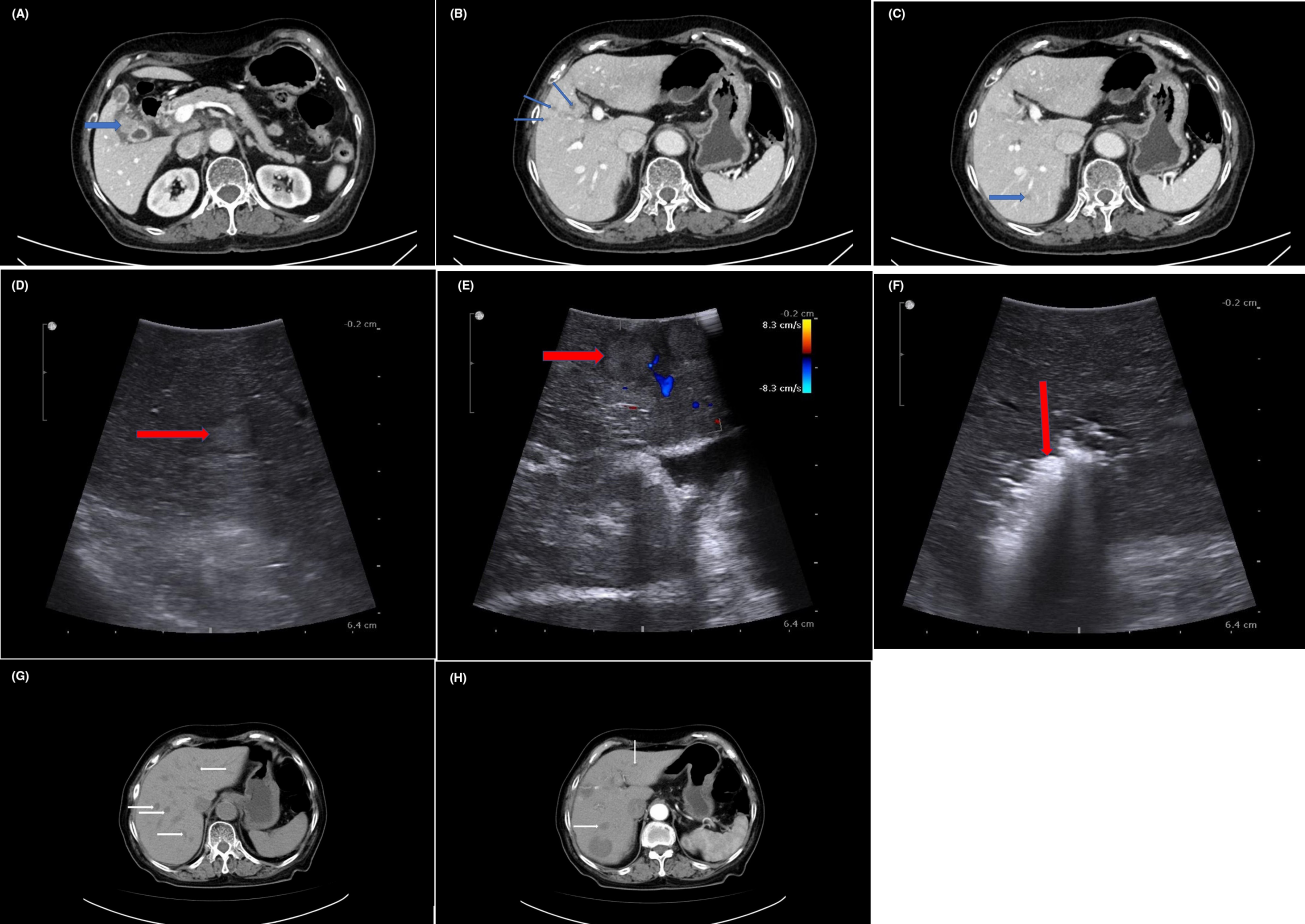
Representative figures of GBC patients with CTMLD. (A) primary gallbladder lesion (blue arrow); (B) regional metastatic liver lesions (blue arrow); (C) distant isolated metastatic liver lesion; (D) and (E) metastatic liver lesions detected by intraoperative ultrasound; (F) intraoperative radiofrequency ablation. The patient finally received a gallbladder excision, partial hepatectomy (SIVB + V), a regional lymphadenectomy, and a combined radiofrequency ablation (S7 metastatic lesion). Nearly 2 months after surgery, recurrent disease were detected within liver (G) and (H) (white arrow). CTMLD, countable and treatable metastatic liver disease; GBC, gallbladder carcinoma.
